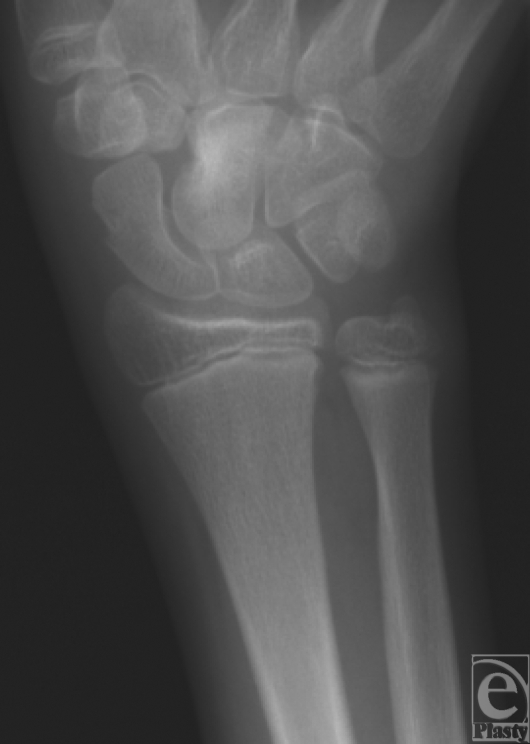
Original radiograph of the left wrist.
